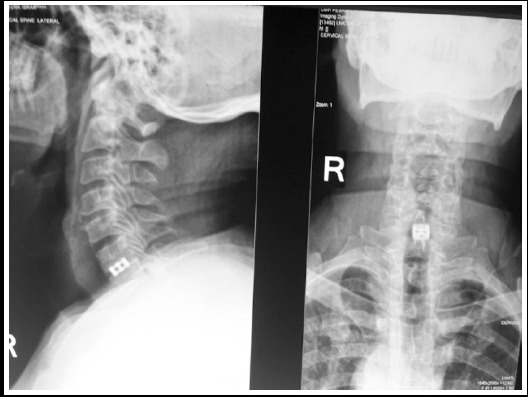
Fusion with titanium cage on cervical X-ray at C6/C7 spinal level at 1 year of follow up.
